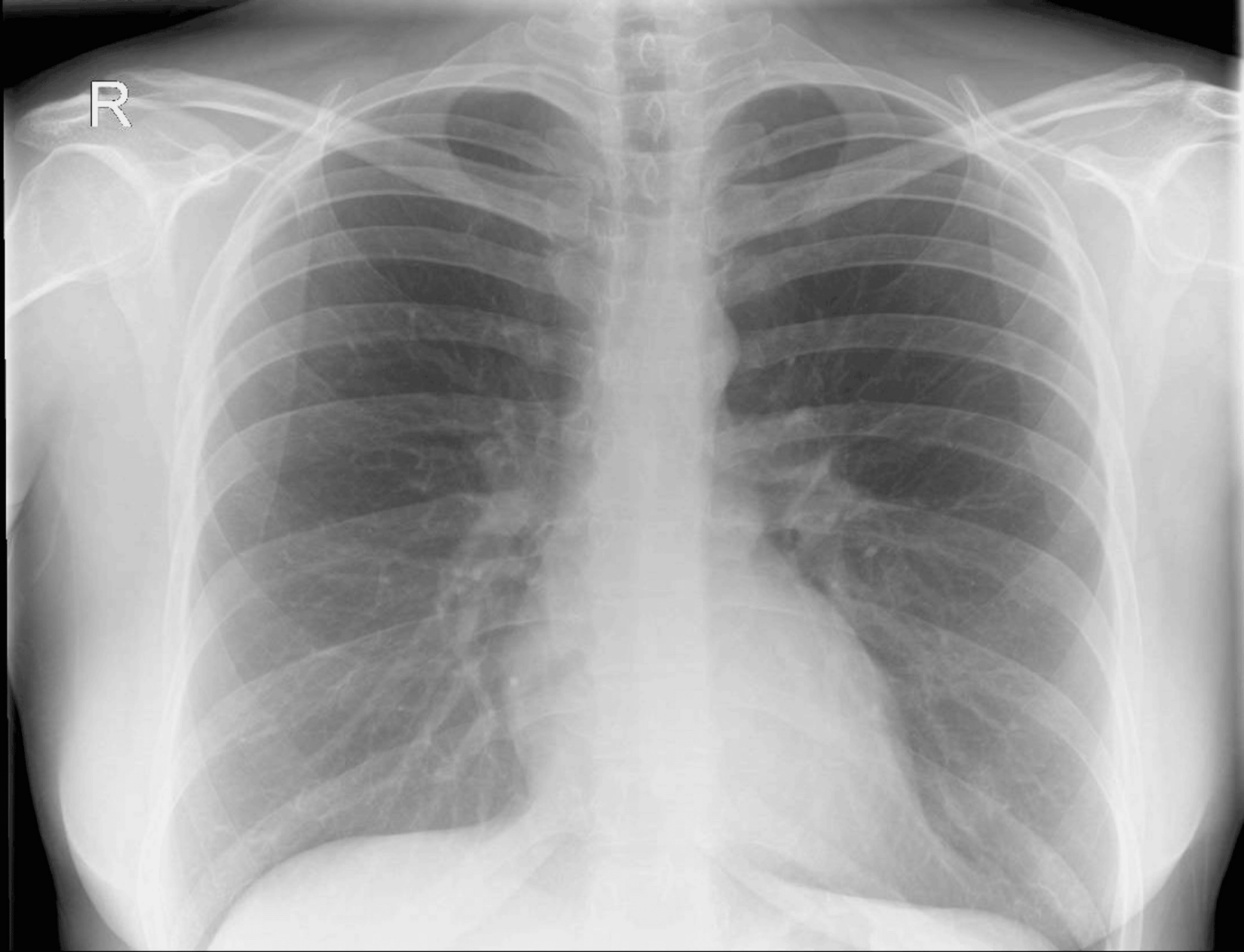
Chest X-ray posteroanterior view with an unremarkable appearance and clear apices, making a Pancoast tumor very unlikely.
